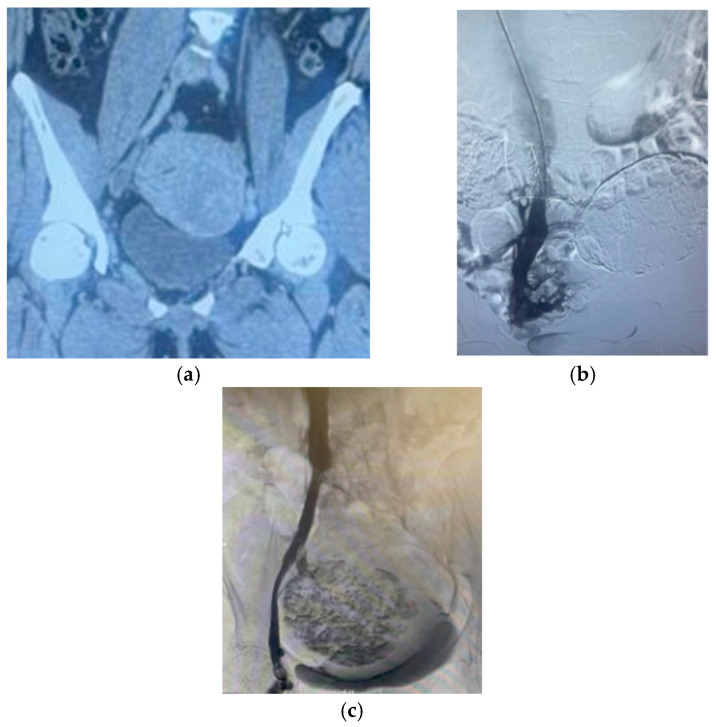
(a) CT Venogram showing a large uterine fibroid compressing the right external iliac vein complicated by DVT. (b) Patient with a large calcified uterine fibroid causing DVT and chronic occlusion of the left iliac veins. (c) Pelvic venogram in a patient with CTEPH showing large calcified uterine fibroid causing right external iliac compression.
